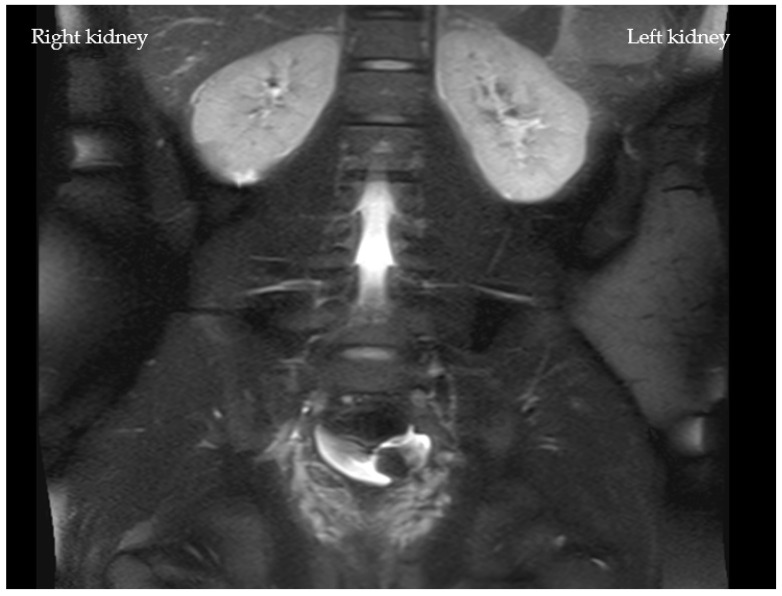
MRI of the abdomen of Case I° with a frontal cut of the two kidneys. An MRI of the abdomen showed a mildly enlarged appendix without surrounding edema and little free fluid in the pelvis. The kidneys showed mildly irregular signaling in the upper renal pole on both sides and the lower pole on the right side (cortical). Duplex sonography of the renal artery showed a laterally symmetrical resistance index normal for age.
